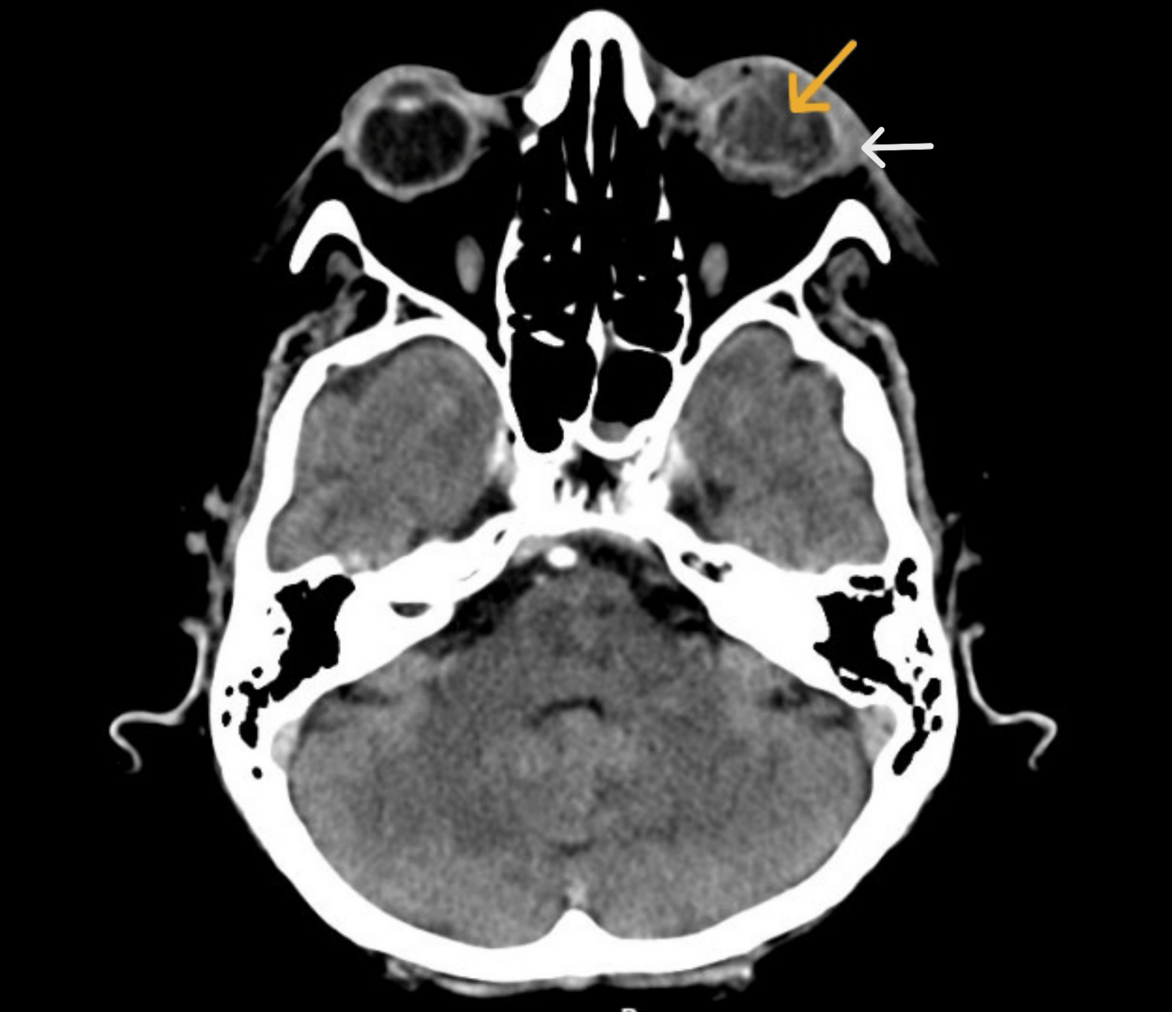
Axial computed tomography showing thickening of the left globe wall (white arrow) and heterogeneous vitreous content (orange arrow).
